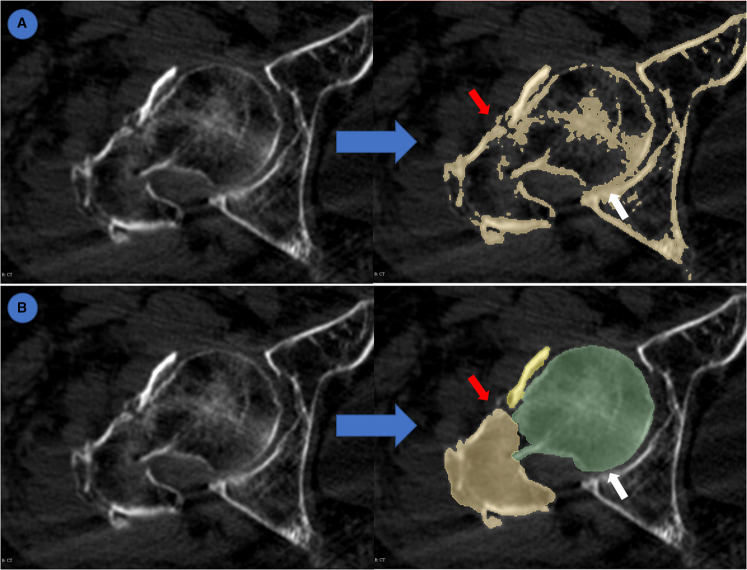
Evaluation of semantic segmentation and threshold-based segmentation. (A) threshold-based segmentation; (B) semantic segmentation. Blue arrow: from original CT to segmented masks. Red arrow: different fracture fragments were highlighted by different colors which made it easy to distinguish. White arrow: adhesion between pelvis and femur.
